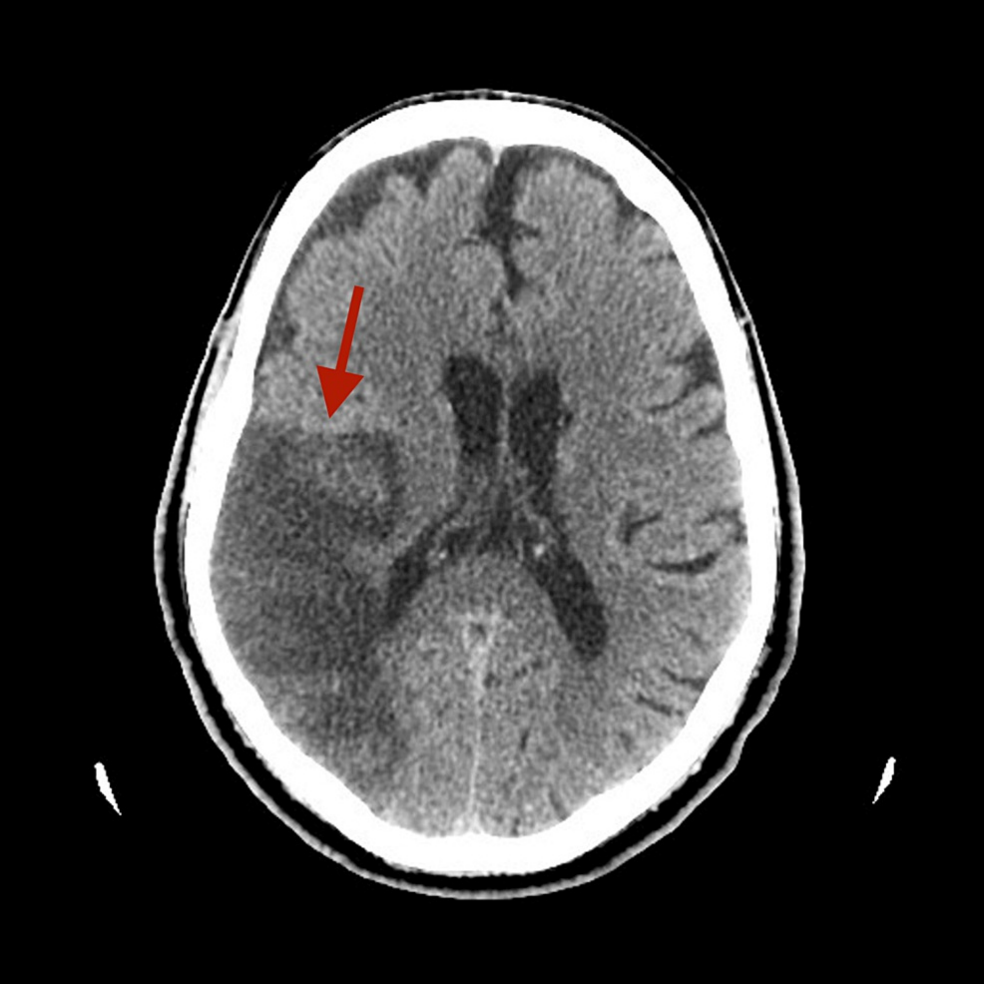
Follow-up non-contrast CT scan on day four revealing expansion of the right middle cerebral artery (MCA) stroke.
